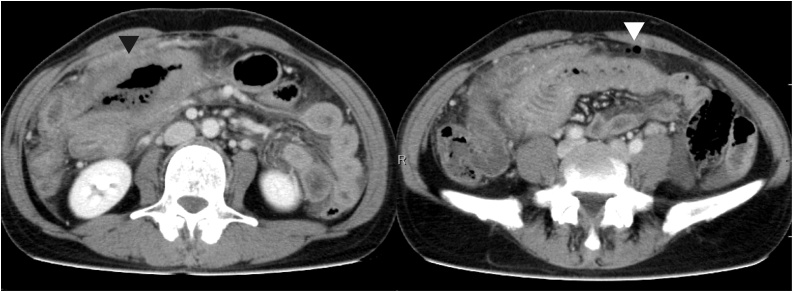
An abdominal computed tomography scan showing thickening of the proximal jejunum wall and the presence of free air in the peritoneal cavity, near the jejunum.
